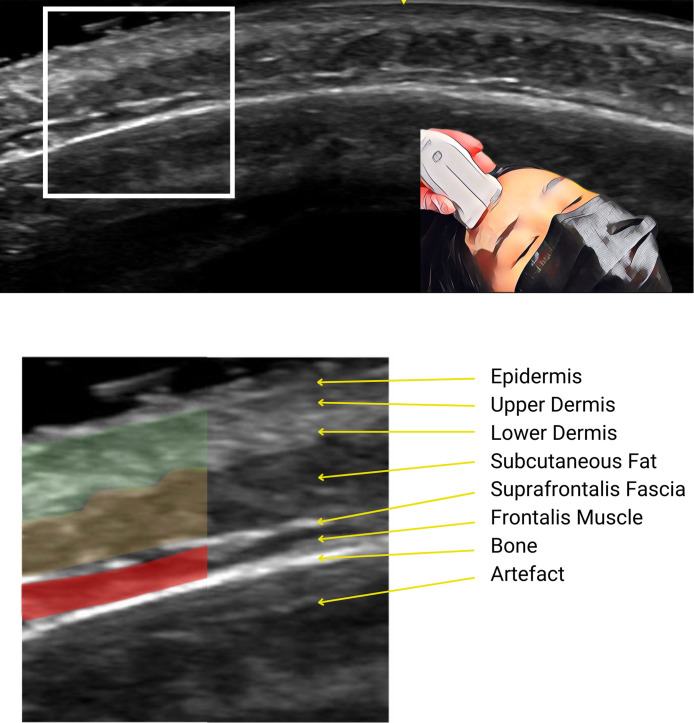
Forehead ultrasound anatomy, of a 24-year-old female, performed with the probe positioned as shown in the inset. Tissue layers are identified in the lower image, with each color representing a distinct tissue layer (green, upper and lower dermis; orange, subcutaneous fat; red, frontalis muscle).
